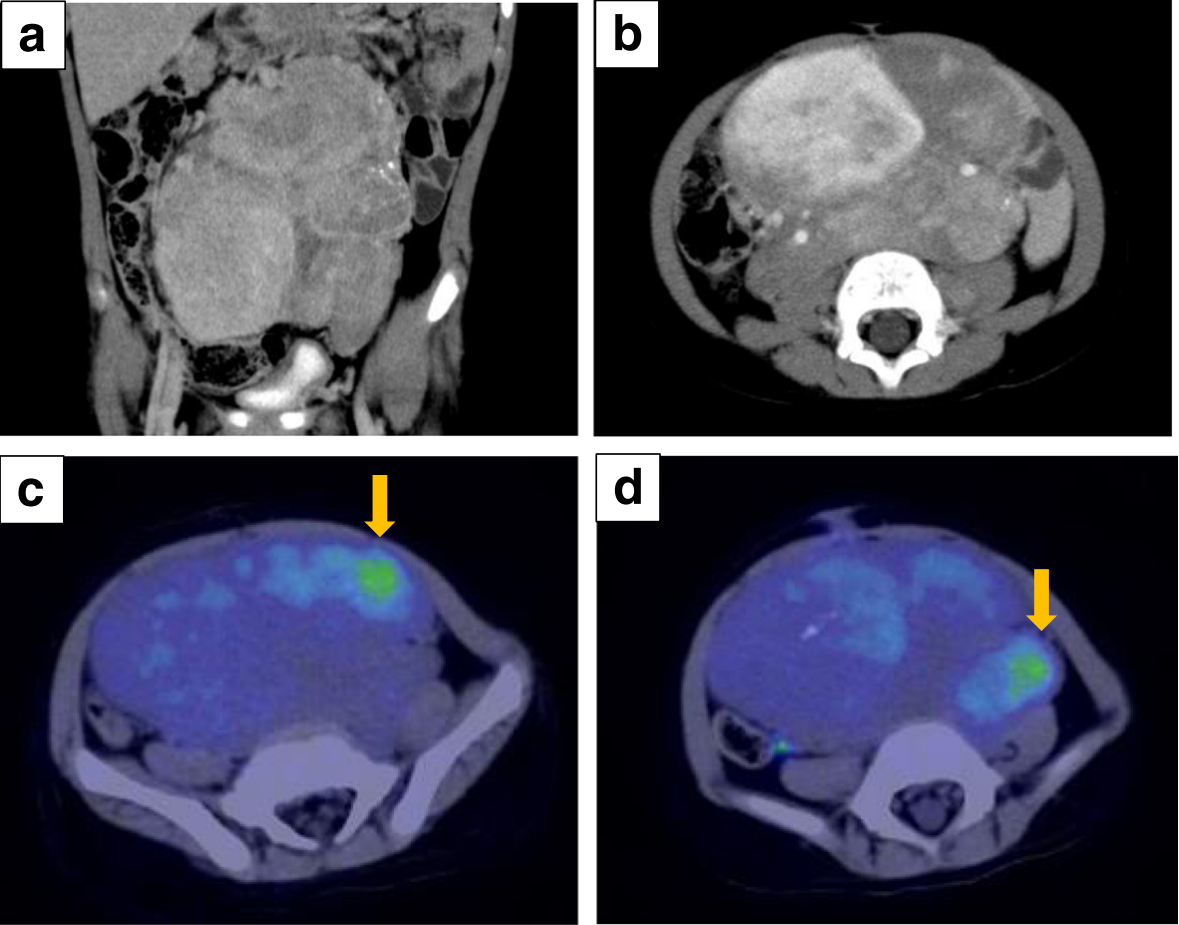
a A coronal computed tomographic image showing a huge abdominal tumor extending from the retroperitoneum to the pelvis. b Axial contrast-enhanced computed tomography of the abdomen showing heterogeneous enhancement. The tumor shows bilateral encasement by the common iliac artery. c and d Axial fluorodeoxyglucose positron emission tomography/computed tomography showing abnormal accumulation with a maximum standardized uptake value of 4.20 (*arrows*)
